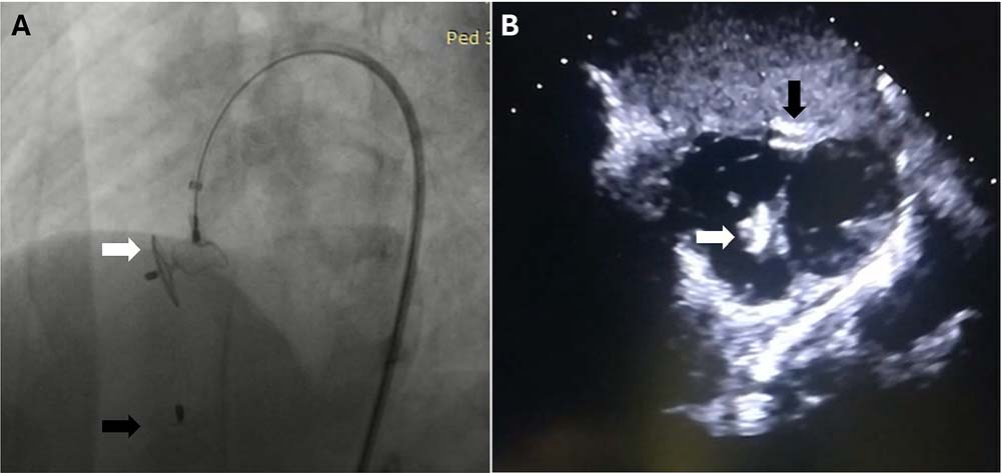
Post-procedural imaging demonstrates successful device placement. (A) Angiogram showing two well-positioned devices at the right ventricular apex (black arrow) and ventricular septum (white arrow). (B) Two-dimensional echocardiographic image confirming optimal device positioning at the right ventricular apex (black arrow) and ventricular septum (white arrow) with no residual leakage.
